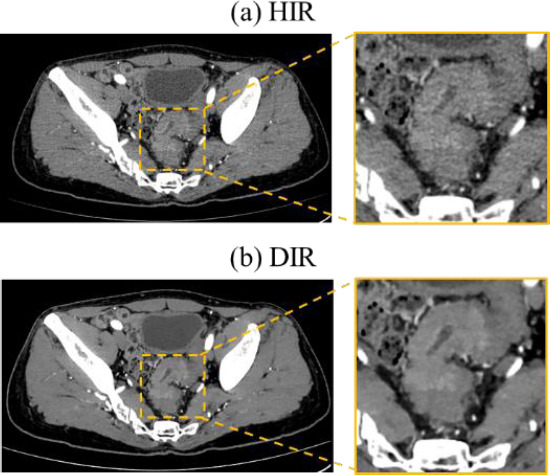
Contrast-enhanced abdominopelvic CT obtained at the arterial phase in a 71-year-old man with colorectal cancer. Images were acquired at a CTDI_vol_ of 12.04 mGy and reconstructed with HIR (a) and DIR (b). While the routine-dose HIR image is acceptable for the detection of colorectal cancer, the presence of image noise and streak artifacts in the pelvic region hindered the differentiation of the tumor from the surrounding tissue. DIR, in contrast, improves image sharpness and lesion conspicuity, better delineating the tumor boundary and enhancement pattern.
